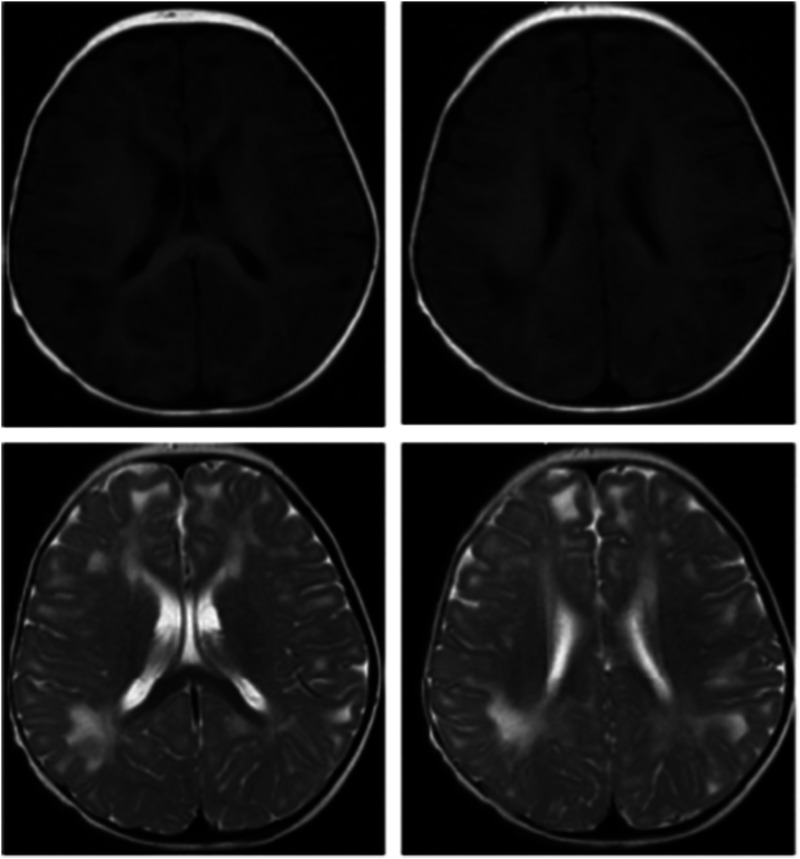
Brain magnetic resonance imaging (MRI) scan of the proband. The axial image using T2-weighting demonstrates bilaterally high-intensity white matter that is abnormal in the centrum semiovale. This image displays a tigroid pattern with a radial arrangement of hypointense stripes, clearly indicated by the arrows. Importantly, the white matter of the subcortical areas remains unaffected. The FLAIR image in the axial plane displays an extensive region of hyperintense white matter within the centrum semiovale. FLAIR, fluid-attenuated inversion recover.
